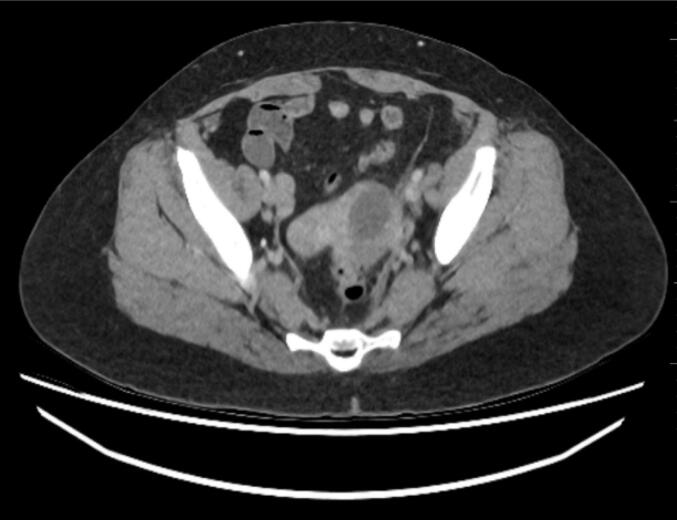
Axial contrast-enhanced CT demonstrating thick-walled cyst in the left ovary.
